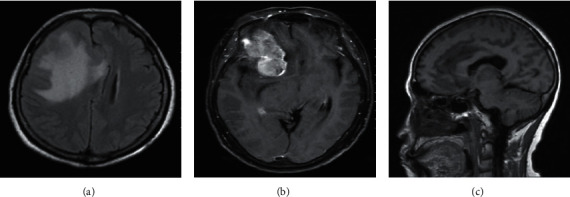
MRI image of a patient with meningeal melanoma (male, 52 years old, with irregular tumor on the left side of the temple). (a) The patient shows uniform low signal intensity on axial T2WI, and there is no edema around the tumor. (b) Axial T1WI shows uniform high signal intensity. (c) Axial T1WI display the tumor is significantly enhanced, and the boundary of the lesion is clear.
